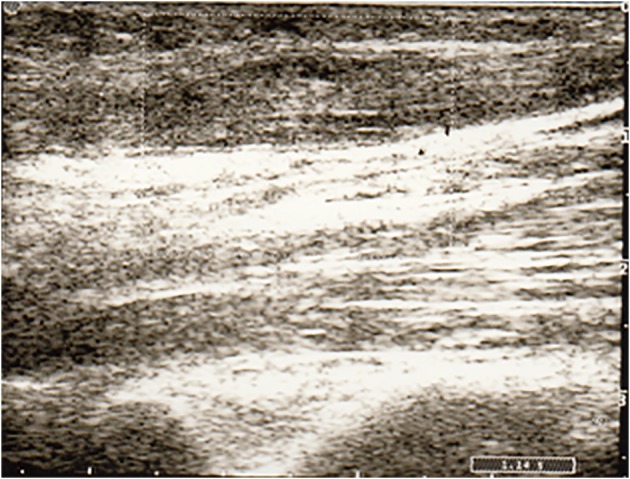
Color-Doppler scan of the right arm showing an enlarged cephalic vein, with echoic material in the lumen and absence of flow signals.
